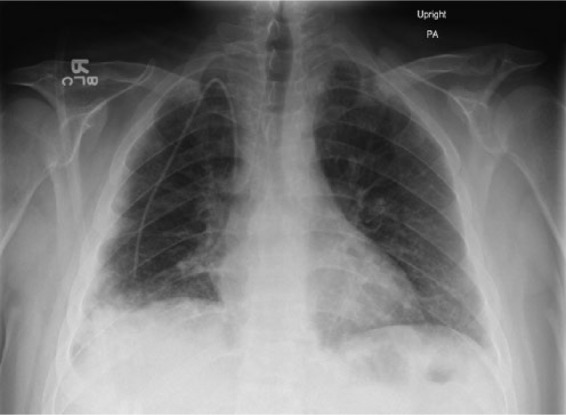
Admission chest X-ray of Patient 2. Bilateral nodular densities, most prominent in the periphery.
